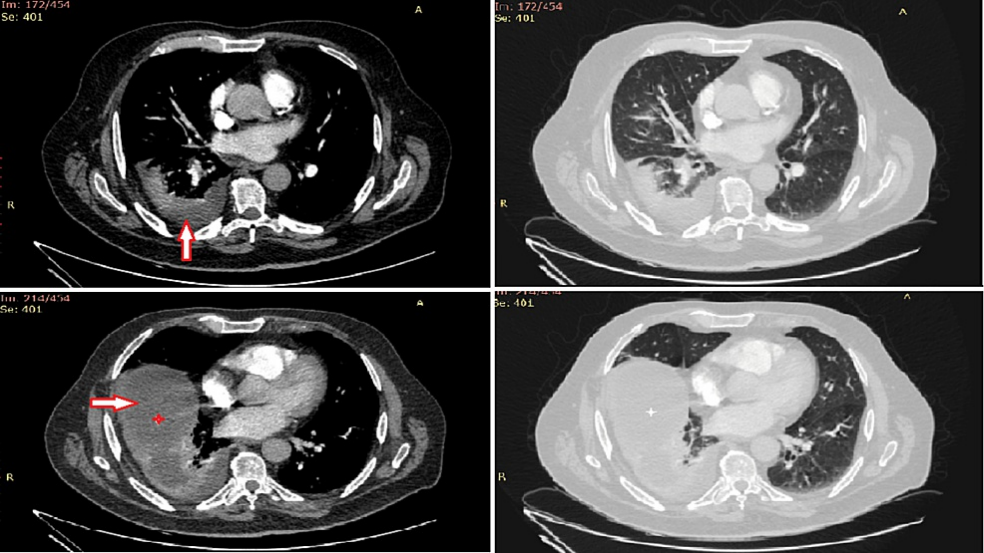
Computed tomography pulmonary angiogram images The arrow points to the right pleural effusion and partial atelectasis of the right lower lobe. In the lower segments of the scan, the subcapsular liver lesion is marked with a star.
